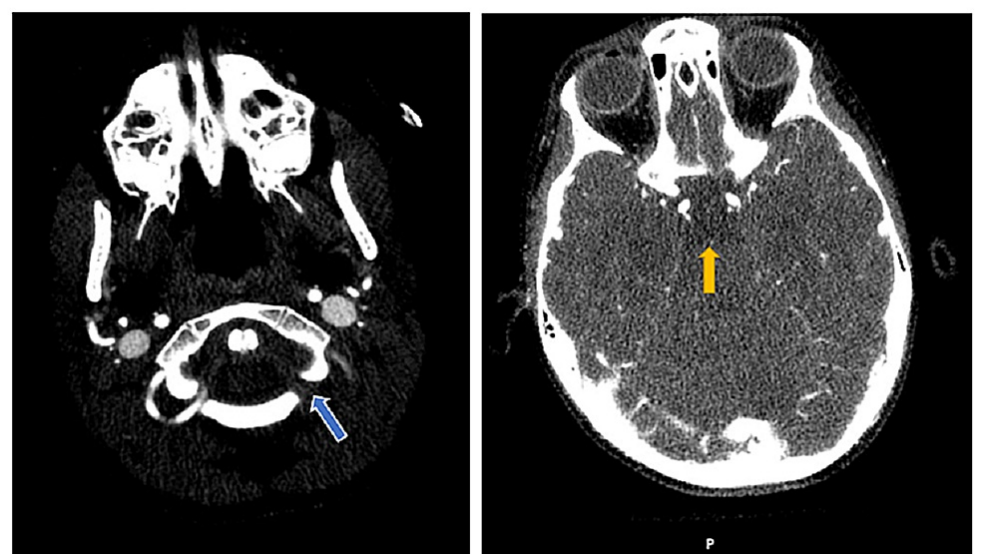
CTA showing the left vertebral artery dissection with associated complete occlusion of left V3 segment of VA (blue arrow) and distal basilar artery thrombosis (yellow arrow). CTA, Computed tomographic angiogram; VA, vertebral artery.
